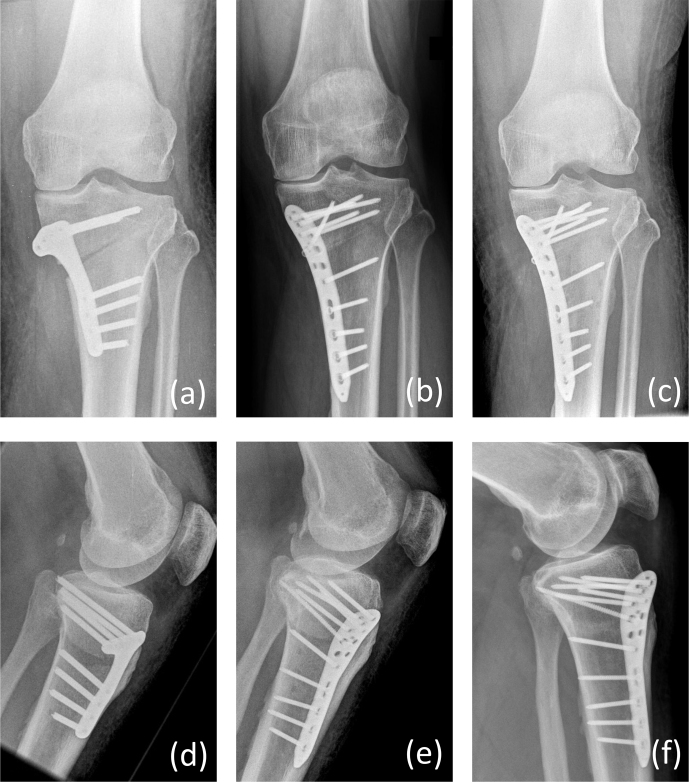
Anterior–posterior (a–p) radiograph on postoperative day 2 after primary osteotomy and osteosynthesis by a plate fixator (a), radiograph on postoperative day 2 after implant exchange and osteosynthesis by a silver-coated plate fixator (b), and radiograph 1 year after revision surgery with complete healing of the osteotomy (c). Additionally, lateral views were shown in the same order as the (a–p) ones (d–f).
